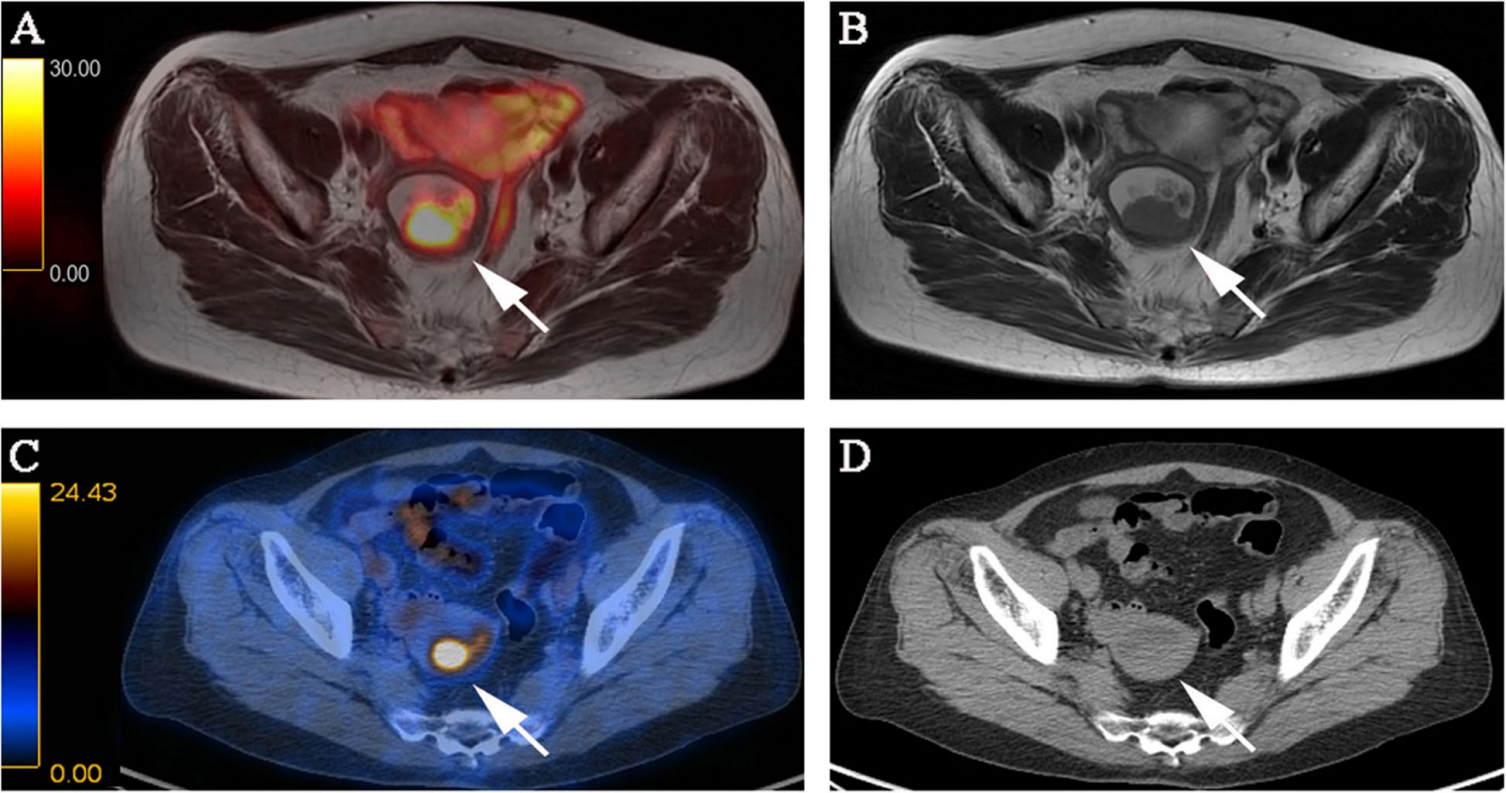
FIGO stage IB, pathologically confirmed as highly differentiated adenocarcinoma in a 64-year-old female patient. A PET/MR images. B MR T2-weighted images. C PET/CT images. D CT images. PET/MRI and MRI show the endometrial carcinoma invading > 50% of the myometrium. PET/CT shows the endometrial carcinoma invading < 50% of the myometrium, while CT is difficult to show the border of the tumor. The PET/MRI staging was consistent with the pathological stage, namely FIGO stage IB. The PET/CT staging was IA. The white arrow indicates endometrial carcinoma. FIGO, International Federation of Gynecology and Obstetrics; PET, positron emission tomography; MRI, magnetic resonance imaging; CT, computed tomography
